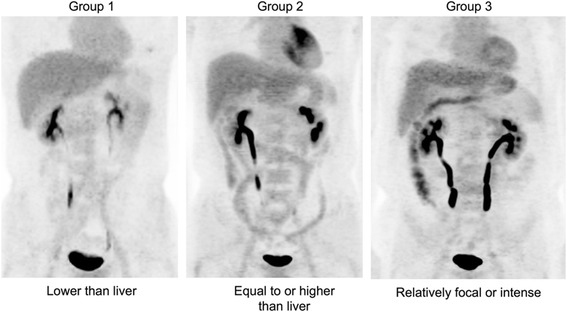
Intestinal ^18^F-FDG uptakes classified by visual analysis
